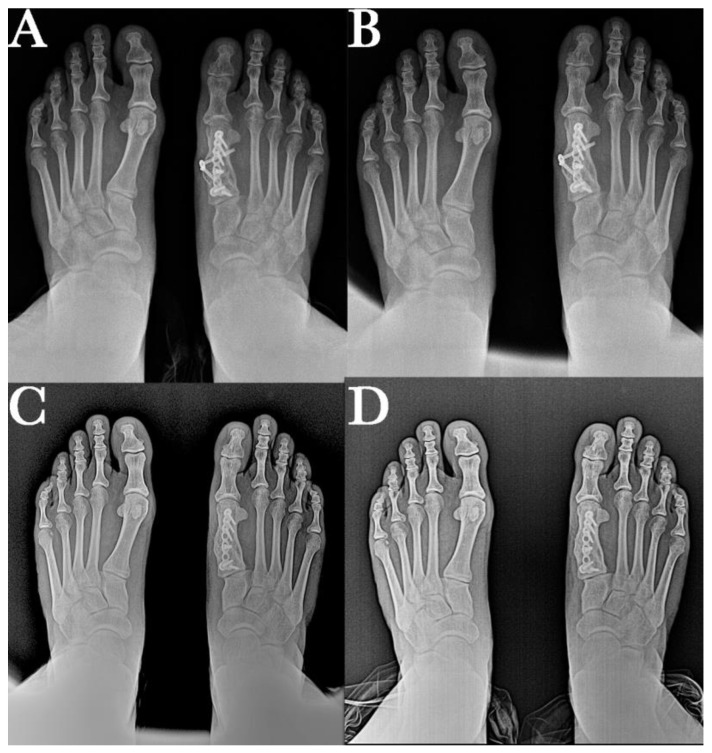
Correct position of the first metatarsal in the sagittal plane at (A) 6-month, (B) 12-month, (C) 18-month, and (D) and final 24-month follow-up after surgery, showing bone callus consolidation and remodeling, and maintaining correction of the analyzed radiographic parameters.
